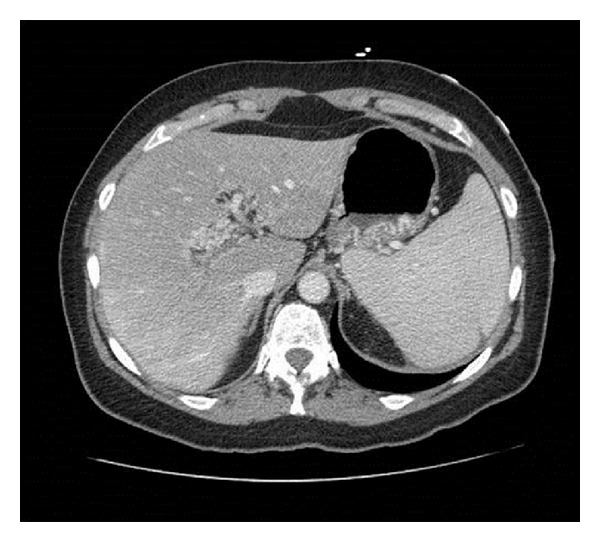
CT demonstrated periportal collateral circulation and gastric varices.
